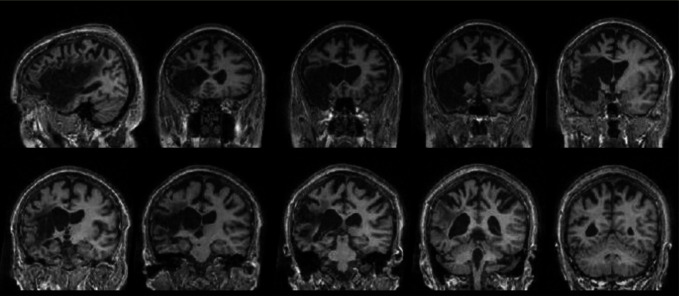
MRI of SKO acquired 9 years post-stroke, demonstrating an extensive left fronto-temporo-parietal lesion. Presented are a single sagittal slice, with nine coronal slices from anterior to posterior through the lesion area.
